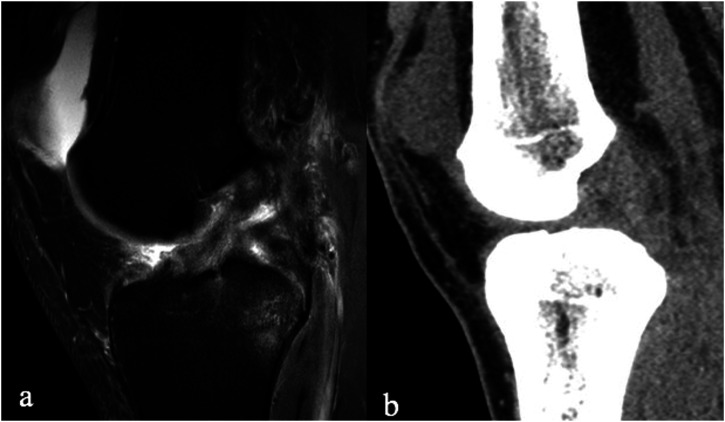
True positive. A 24-year-old male with an arthroscopy verified torn ACL in both imaging modalities. Sagittal MRI (PD, TE 20 ms., TR 1800 ms., slice thickness 3 mm) (a) and sagittal DECT (b). The Readers were able to freely adjust window settings and do reconstructions in any desired plane when analyzing DECT images.
